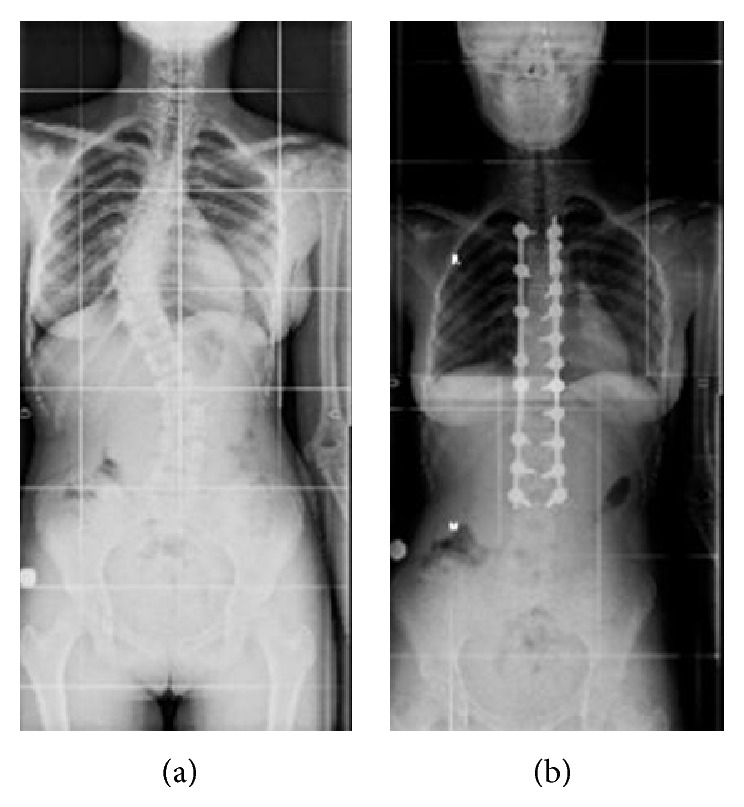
(a) Using preoperative radiography, thoracic scoliosis with right curvature was observed. (b) Postoperative radiography showing complete resolution of the spinal curvature after surgery.
